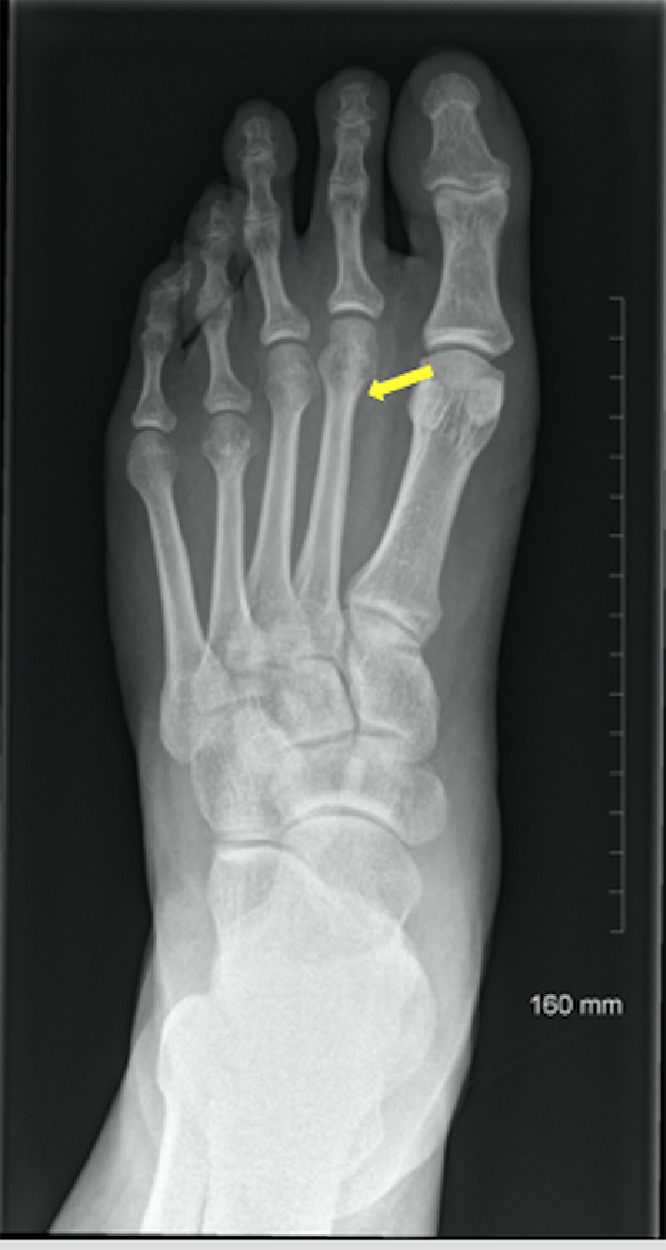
Oblique and DP radiographs of the left foot from Aug 2019, one month post initial presentation. There is very subtle periosteal reaction at the medial aspect of the neck of the second metatarsal (arrows).
